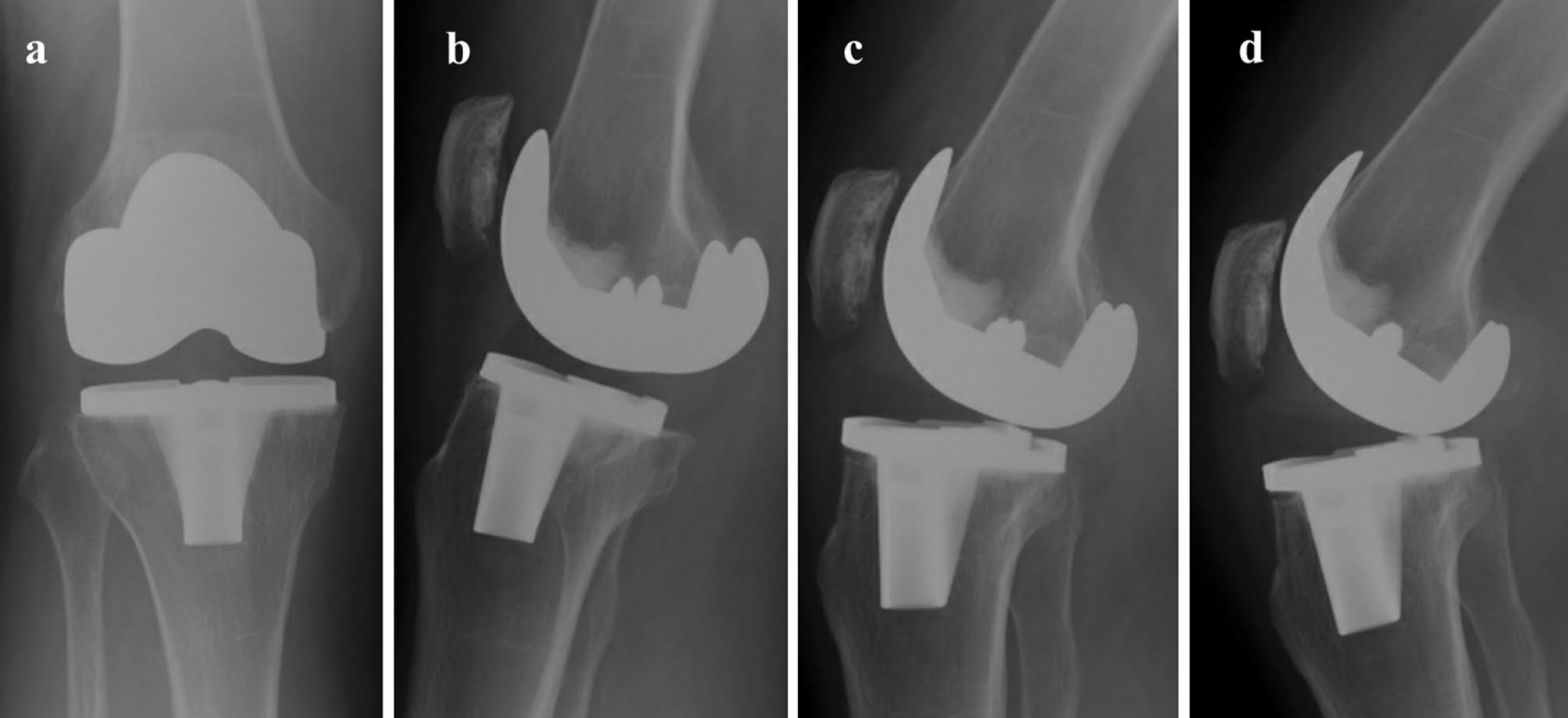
Weightbearing radiographs in (a) Anteroposterior extension (b) lateral extension, (c) lateral 30° flexion and (d) lateral 45° flexion weightbearing radiographs of knee. No definite polyethene thinning is evident.
